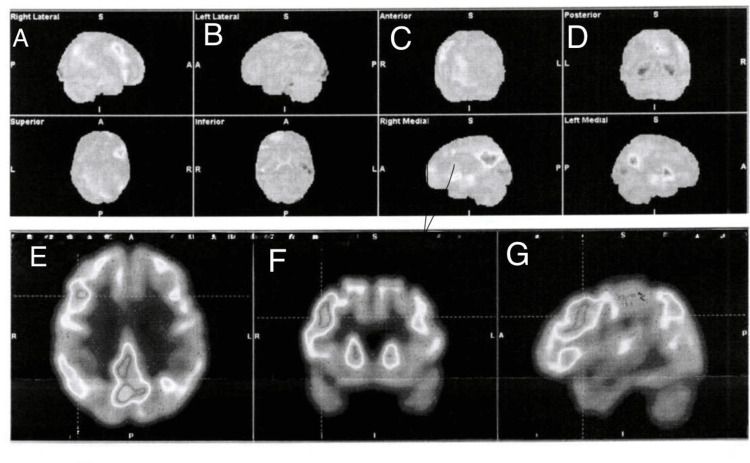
Brain fluorodeoxyglucose positron emission tomography (FDG-PET). Brain FDG-PET demonstrates a pattern of regional metabolic abnormalities consistent with an active inflammatory encephalitic process. Panels A–G correspond to three-dimensional cortical surface projections, including right lateral (A), left lateral (B), anterior (C), posterior (D), superior (E), inferior (F), and right medial (G) views. The study reveals marked hypermetabolism in the right frontal lobe, predominantly involving the superior frontal gyrus and frontobasal regions, as well as bilateral hypermetabolism of the posterior cingulate cortex, findings suggestive of focal cortical hyperexcitability and active neuroinflammation. In contrast, reduced metabolic activity is observed in the bilateral temporal and occipital cortices, with left-sided predominance, along with focal hypometabolism in the left parietal region, a pattern consistent with cortical dysfunction secondary to inflammatory involvement. Metabolic activity is preserved in the basal ganglia and thalami, with mild asymmetry in cerebellar metabolism. The combination of frontal–cingulate hypermetabolism and posterior cortical hypometabolism, in the absence of corresponding structural abnormalities on conventional neuroimaging, is characteristic of autoimmune encephalitis and supports an active inflammatory encephalitic process.
